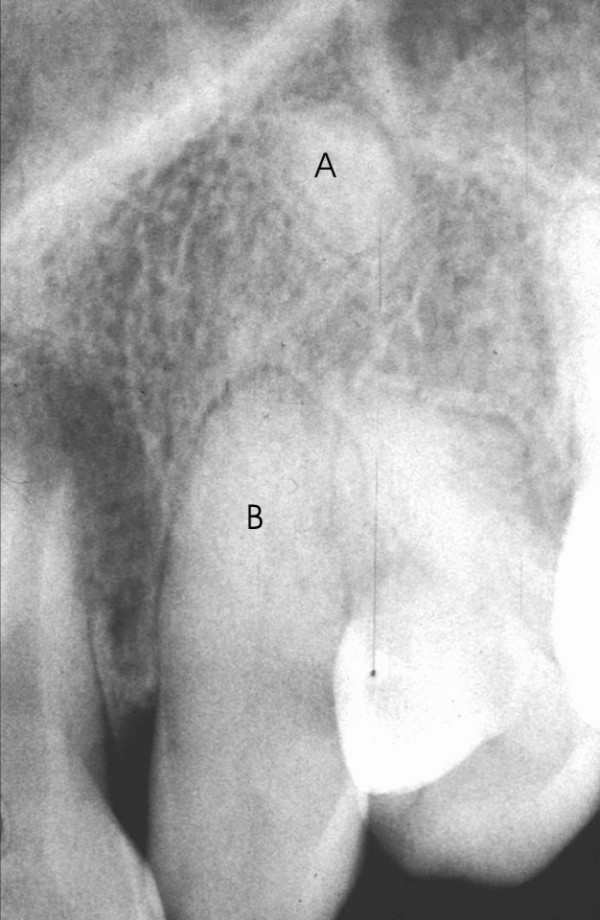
Periapical radiograph after the complete exposure of the tooth crow in the oral cavity and position in the dental arch. A. The apical fragment remains close to its preoperative position; B. The pulp root canal is almost completely obliterated.
